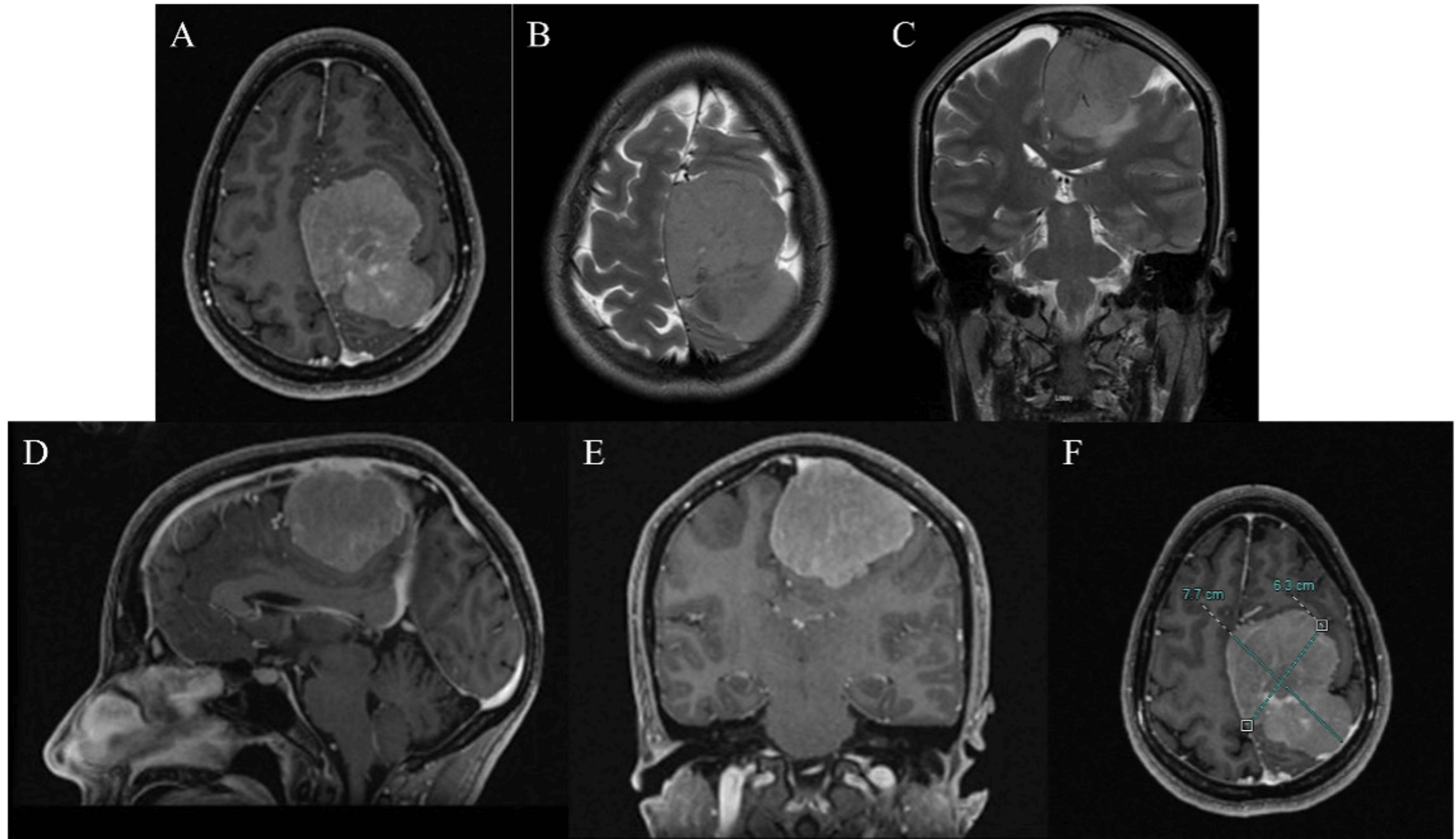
Brain MRI images show hyperintensity in the left frontoparietal lobe that enhances with contrast and with evidence of the pia mater on the T2 image and midline shift with mass effect with cingulum herniation and lateral ventricle compression at the level of the corpus callosum body and the splenium of the corpus callosum. (A) Axial MRI shows a giant meningioma that enhances with contrast with mass effect. (B) Axial T2 MRI shows an isointense meningioma with a partial arachnoid plane. (C) Coronal T2 MRI shows a left parasagittal meningioma with edema at the cortical level suggesting parenchymal infiltration and related to the affected motor area. (D) Sagittal T2 MRI shows parasagittal meningioma at the middle third of the superior longitudinal sinus related to the motor and sensitive areas. (E) The coronal view shows a parasagittal meningioma, which enhances with contrast. (F) The axial view shows the larger diameter of the tumoral mass (7.7 × 6.3).
